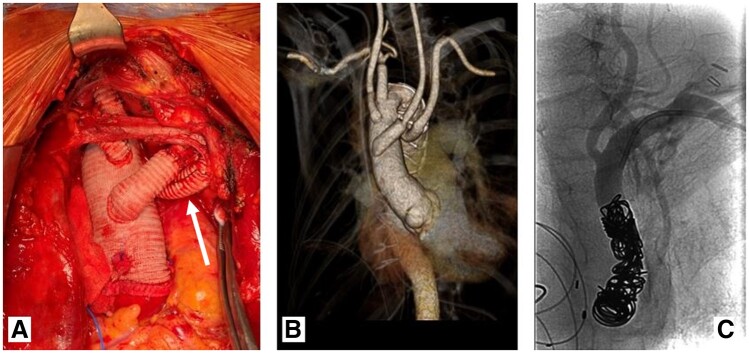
(A) Prosthetic branches anastomosed to right subclavian artery, right common carotid artery and left common carotid artery, perfusion branch to aberrant left subclavian artery bypass (arrow), (B) postoperative computed tomography and (C) aberrant left subclavian artery angiography after coiling.
